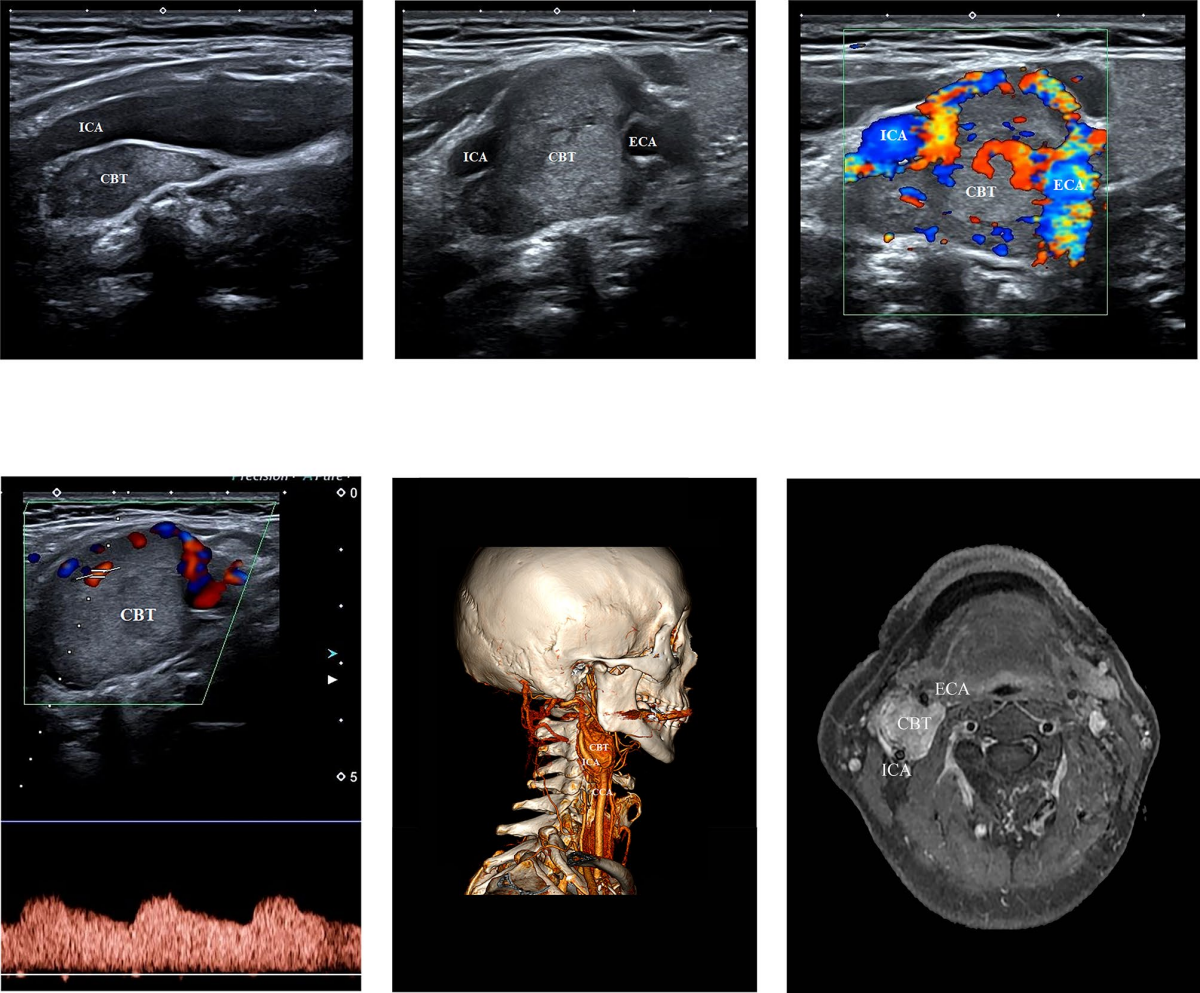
Image data of CBTs were collected on gray-scale, CDU, CTA, and MRA. (a) Gray scale ultrasound image of a Shamblin III CBT on sagittal section and (b) transverse section. Ultrasound imaging detects a clear boundary, solid and hypoechoic mass which located at the carotid bifurcation. (c) CDU images of the CBT with a richly vascular and (d) with inner low-resistance arterial spectrum. (e) Multi slice spiral CTA with 3D VR shows a solid high vascular mass, causing widening of the carotid bifurcation and infiltrating internal carotid artery. (f) MRA images shows the typical separation of the external and the internal carotid arteries of the CBT, with heterogeneous enhancement
